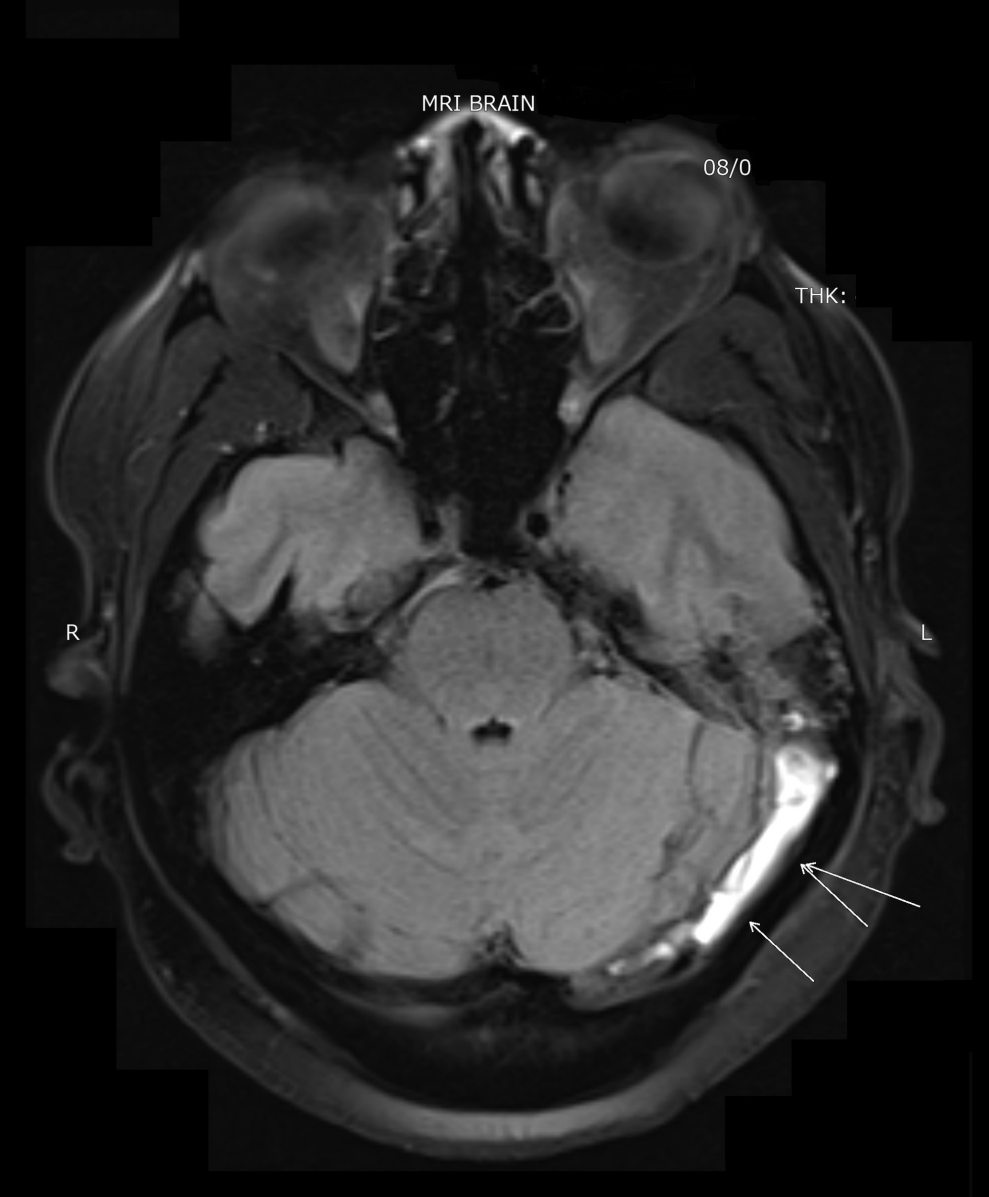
Magnetic resonance imaging of the brain showing thrombosis of the left transverse sinus.
